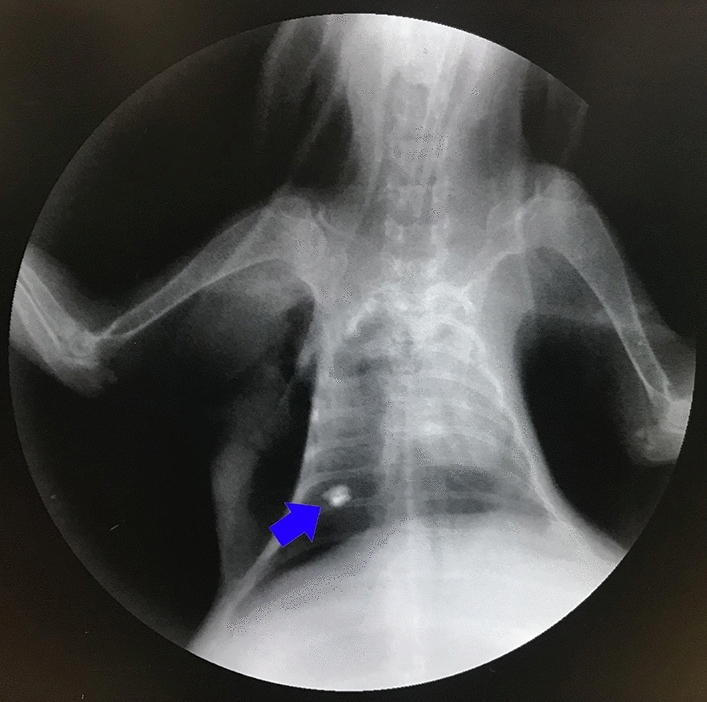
Magnetic gel in lung tissue (blue arrow) observed under X-ray.
